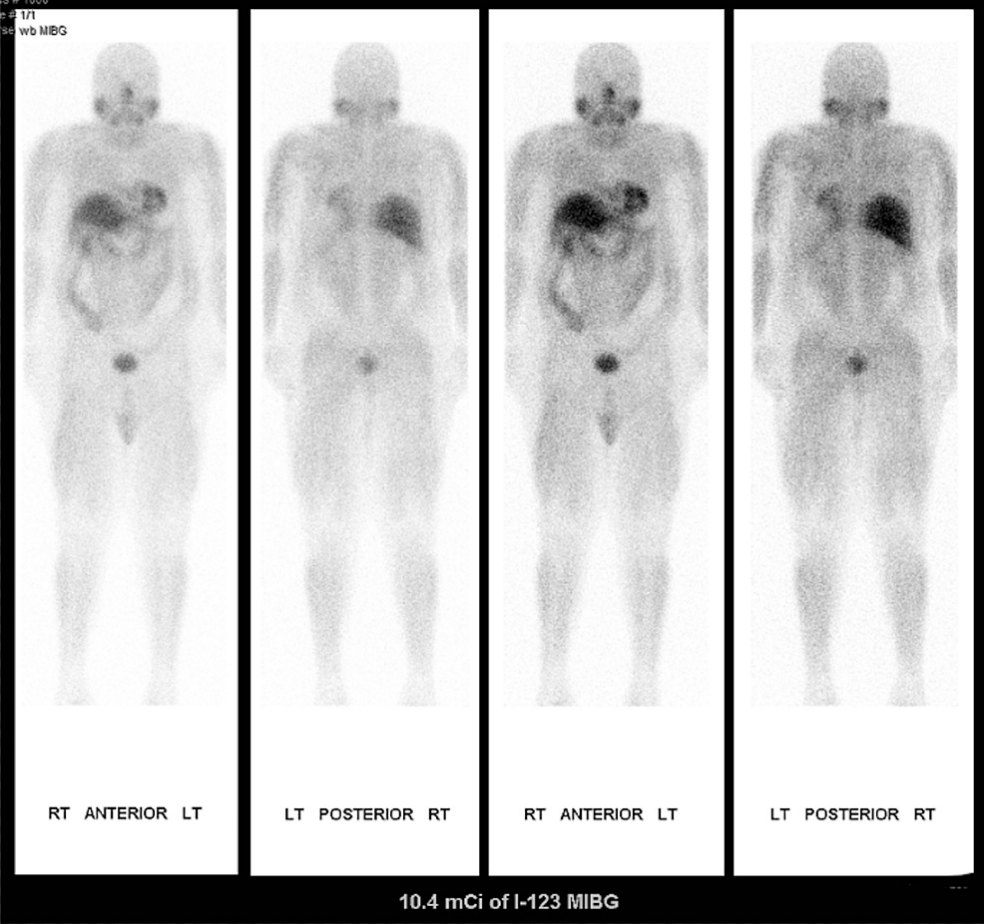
The meta-iodobenzylguanidine (MIBG) scan. Negative whole-body MIBG examination without abnormal radiotracer localization in the left adrenal gland nodule seen on comparison CT examination.
